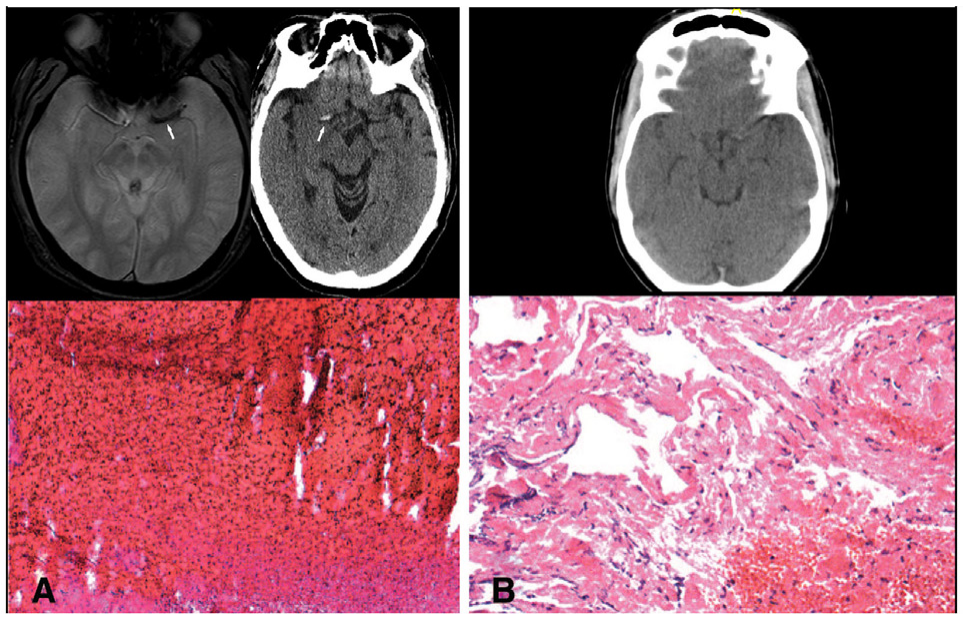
(A) Blooming artifact on gradient echo MRI sequence and hyperdense artery sign on CT with representative red blood cell rich thrombus. (B) Lack of significant hyperdense artery sign with representative fibrin rich thrombus.
